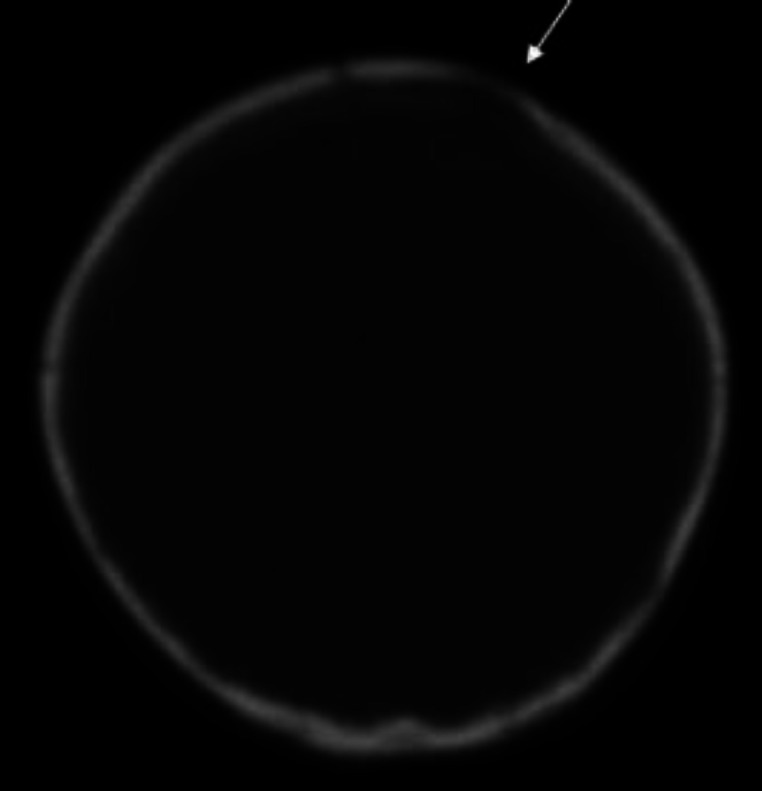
Spiral brain CT scan of a patient: arrow showed a faint lytic lesion in left frontal bone without sclerotic margin.
